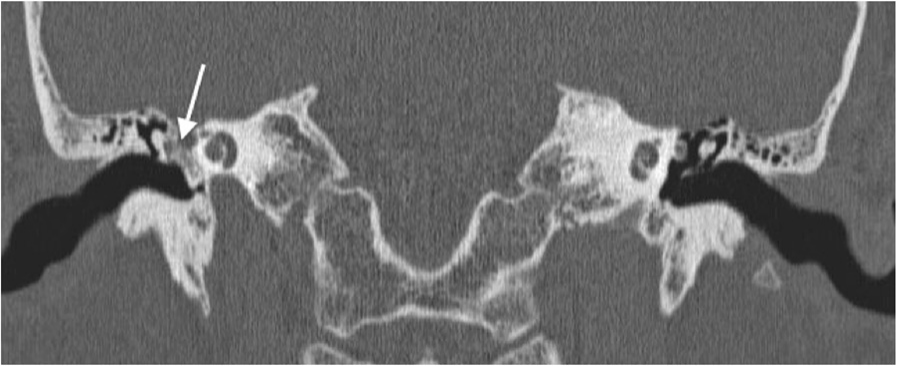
A coronal computed tomographic image showing an osseous lesion in the right middle ear space (arrow).
